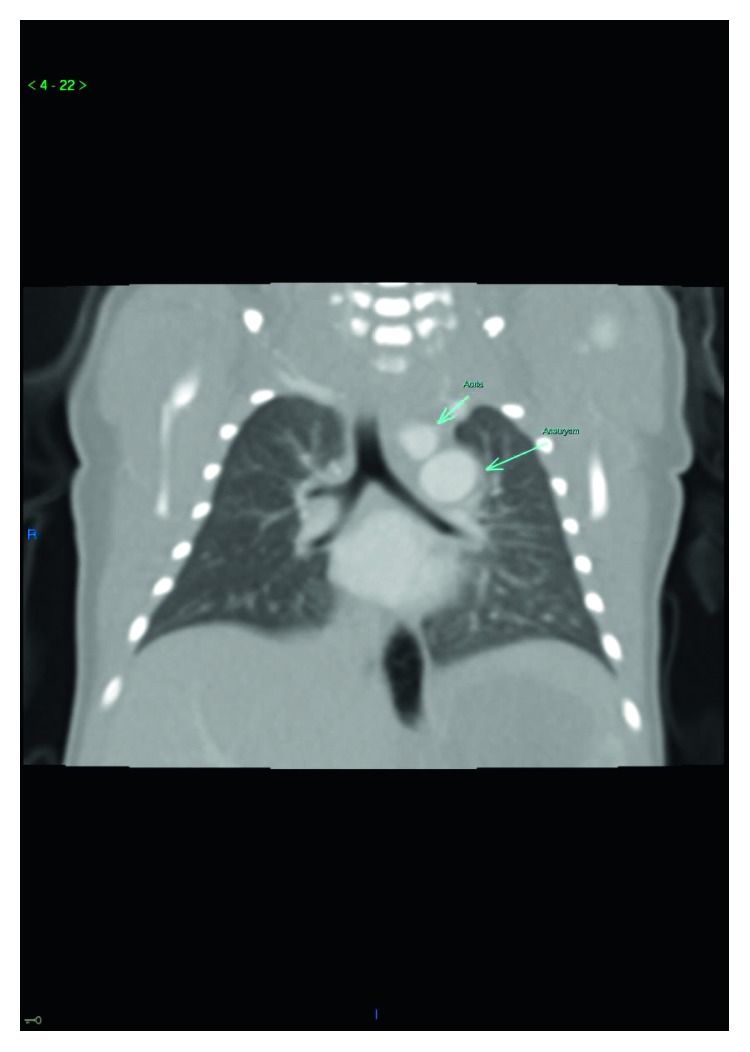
Chest-computed tomography with angiography (CT-A): coronal plane revealing aneurysm relationship to the left bronchus.
